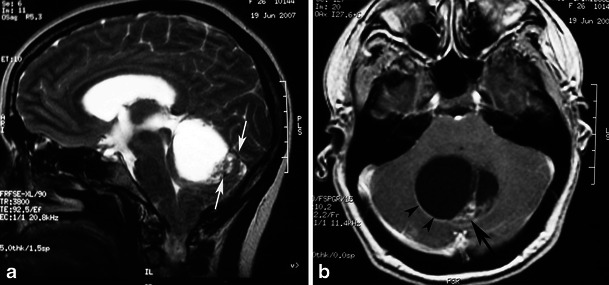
Pilocytic astrocytoma in a 26-year-old woman arising in the vermis. a A sagittal T2-weighted image shows a hyperintense cystic mass of the cerebellar vermis with a peripherally located mural nodule (arrows) compressing the fourth ventricle. b An axial contrast-enhanced T1-weighted image demonstrates intense enhancement of the mural nodule (arrow), as well as cyst wall enhancement (arrowheads)
